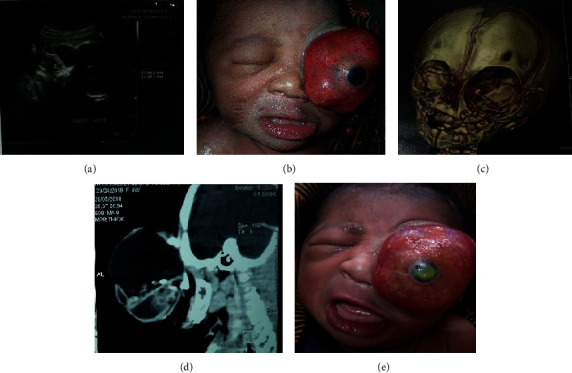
(a) Transabdominal ultrasound of the mother at 36 weeks of pregnancy showing a right orbital mass. (b) Congenital exophthalmia (5 cm). (c) CT scan of the head and the orbits: heterogeneous mass in the left orbit with cystic, calcified, fatty, and vascularized tissues. (d) 3D skull scan: enlarged left orbital cavity. (e) Left eye: corneal abscess with perforation.
